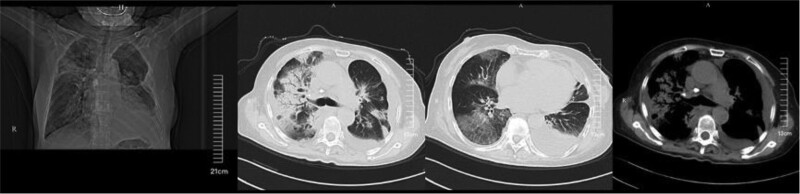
Chest CT before admission. CT = computed tomography.
